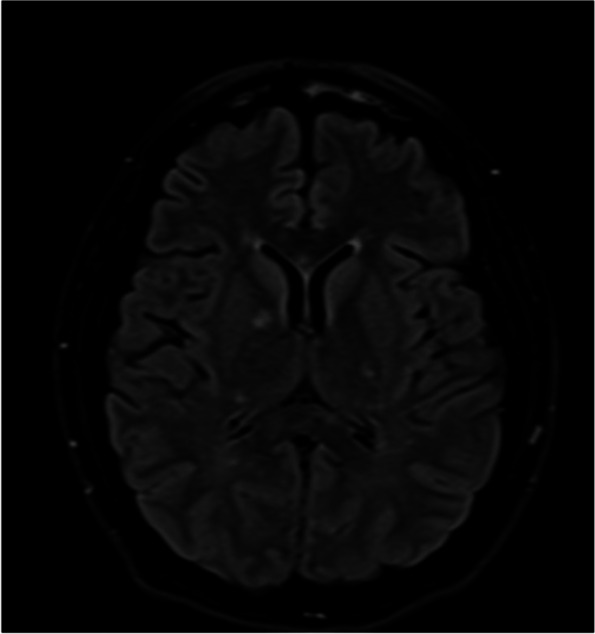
Brain magnetic resonance imaging: T2/FLAIR sequence found small hyperintense nonspecific demyelinating lesions at the periventricular region, internal capsule, and bilateral subcortical areas
